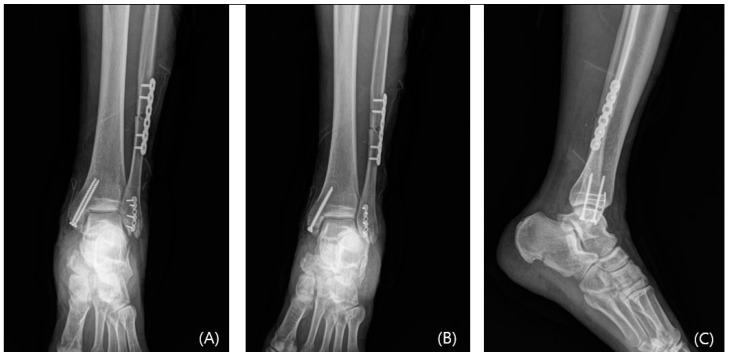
The postoperative mortise, anteroposterior, and lateral radiographs (A–C) showing mini-plate fixation for Wagstaffe tubercle fracture.
